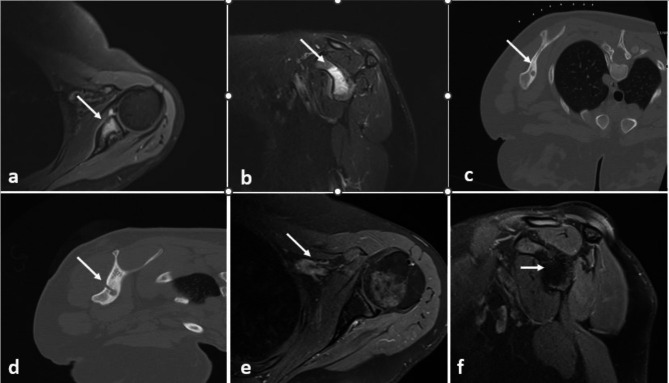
Pre-op axial fat-suppressed T2W a). axial and b). sagittal images, c). pre-op CT axial images, d). post-op fat-suppressed T2W axial images, 3rd month post-op fat surpressed T2W e). axial and f). sagittal images are shown. In the pre-op images, edema is observed within the osteoid osteoma nidus at the level of the coracoid process. In the post-ablation images, it is seen that the edema within the lesion has dissolved, gradually turned into post ablation necrosis and the nidus has disappeared.
